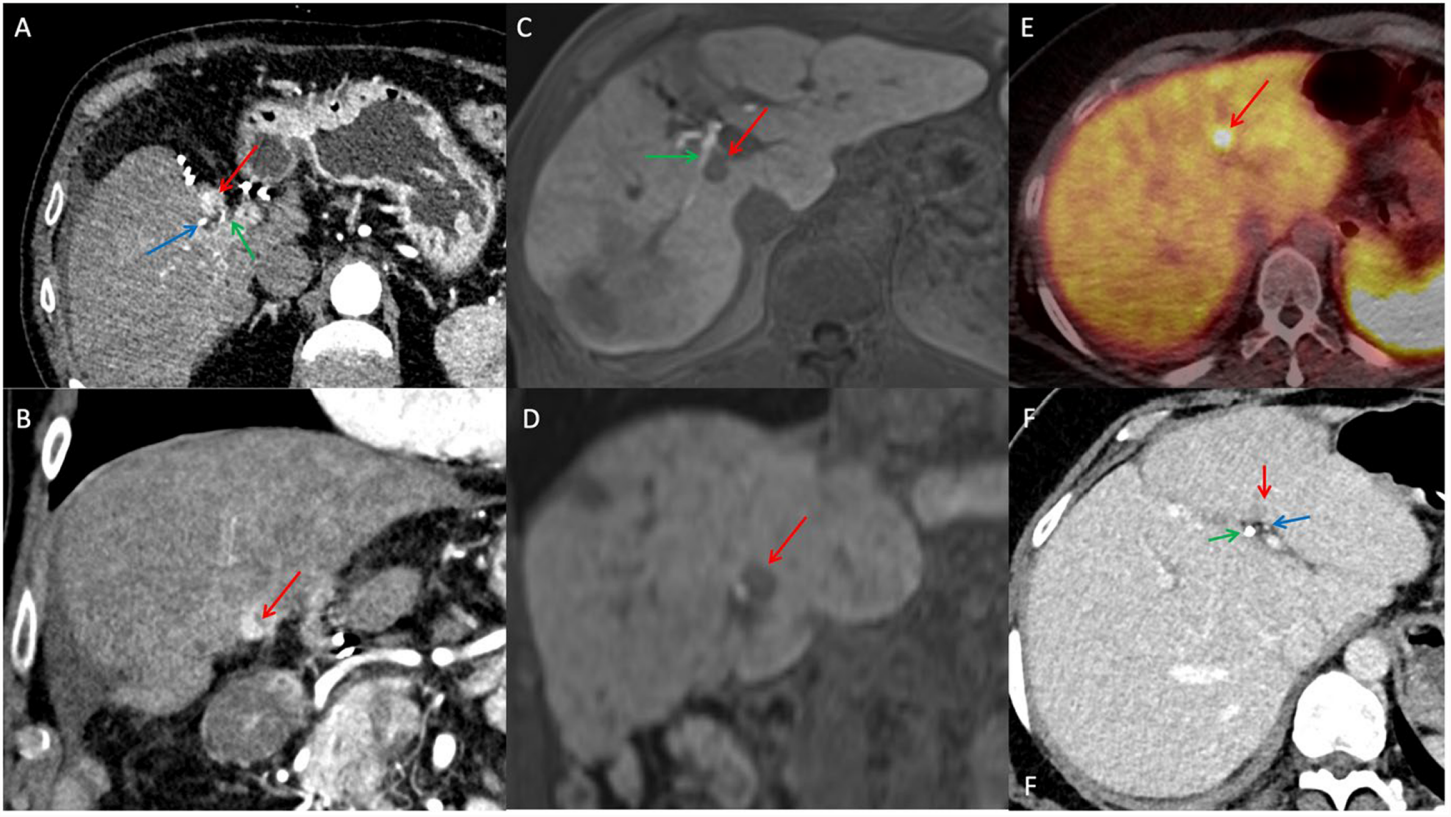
All patients with centrally located liver cancer with pre-interventional imaging before CT-guided cryoablation. Three patients with CLTT are presented: Patient 1 and Patient 2 (A–D) both have HCC adjacent to the right hepatic duct, while Patient 3 (E, F) has a hepatic metastasis of a NET near the left hepatic duct. All patients required extra consideration with protective bile duct measures to minimize the risk of bile duct injury associated with ablation in this central tumor location. Our interdisciplinary tumor board recommended CT-guided cryoablation with bile duct protection via nasobiliary drainage, enabling the perfusion of bile ducts with warm saline during the ablation procedure. *red arrow: tumor; green arrow: bile duct; blue arrow: hepatic artery. *HCC: Hepatocellular carcinoma; NET: neuroendocrine tumor. A + B: CT in axial and coronal orientation (arterial phase). C + D: T1-weighted MRI in axial and coronal orientation (delayed phase). E: PET-CT in axial orientation (Ga-68-DOTA-TATE). F: CT in axial orientation (portal venous phase)
